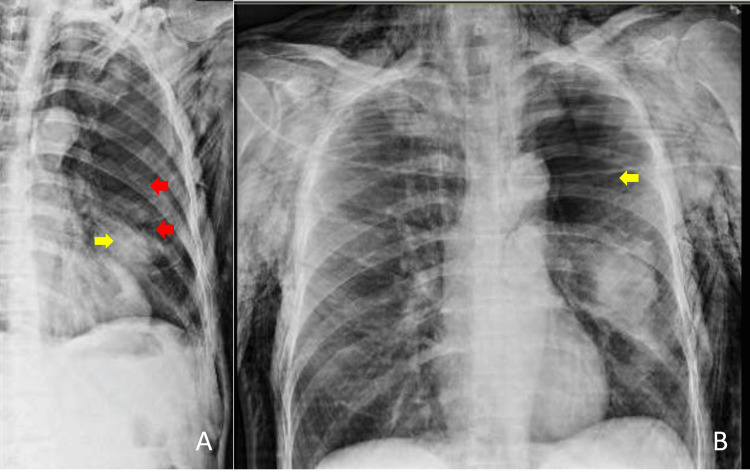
Urgent x-ray of the left rib cage and chest after sudden onset of dyspnea. (A) Pulmonary contusion (yellow arrow) and fractured ribs (red arrows), and (B) obvious subcutaneous emphysema and left pneumothorax (yellow arrow).
